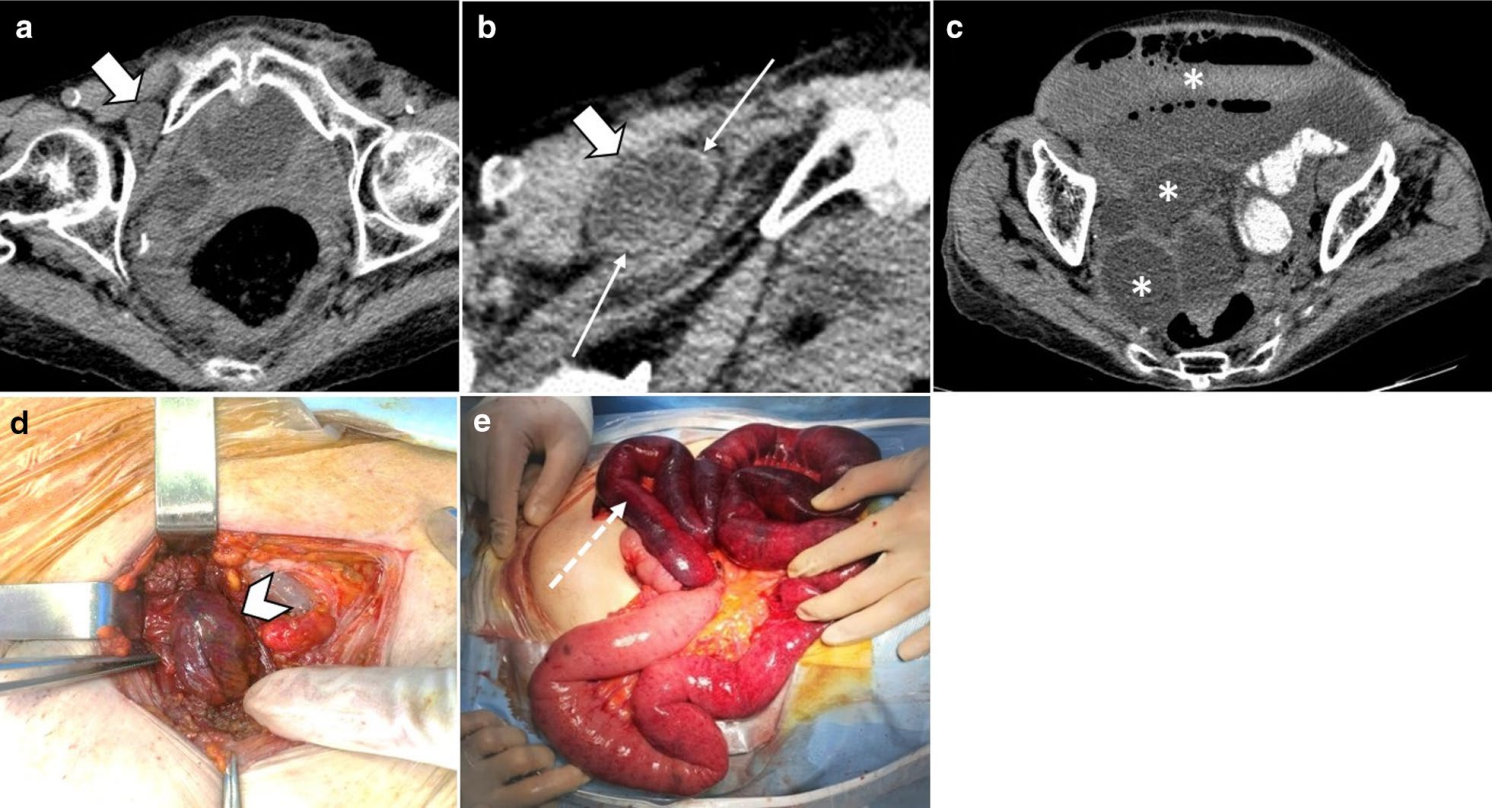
An 84 y/o female with notable abdominal pain and distension for 1 week and obstipation for 2 days. She underwent CT scan with oral and without IV contrast due to elevated serum creatinine level. a–c Right obturator hernia is seen containing a loop of ileum (thick arrow), causing proximal small bowel obstruction (asterisks). No pneumatosis or pneumoperitoneum was found, so the surgeon decided to make a local inguinal incision to reduce the incarcerated ileal loop; however, this was found to be necrotic (arrow head) (d). A subsequent midline incision for laparotomy revealed a long necrotic ileal segment (dotted arrow), that was resected (e). Retrospectively, the herniated loop showed high mural density (thick arrow) on non-contrast CT examination suggestive of hemorrhagic infarct
